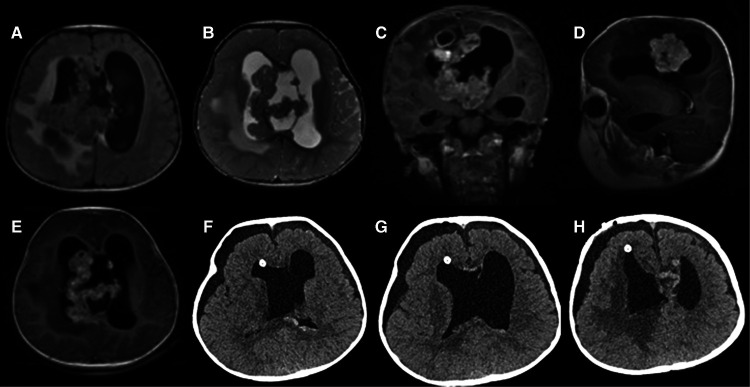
Preoperative MRI: (A) axial T1 image; (B) axial T2 image; (C) coronal T1-weighted image with gadolinium contrast; (D) sagittal T1-weighted image with gadolinium contrast; (E) axial T1-weighted image with gadolinium contrast; Postoperative CT: (F–H) axial scan.
